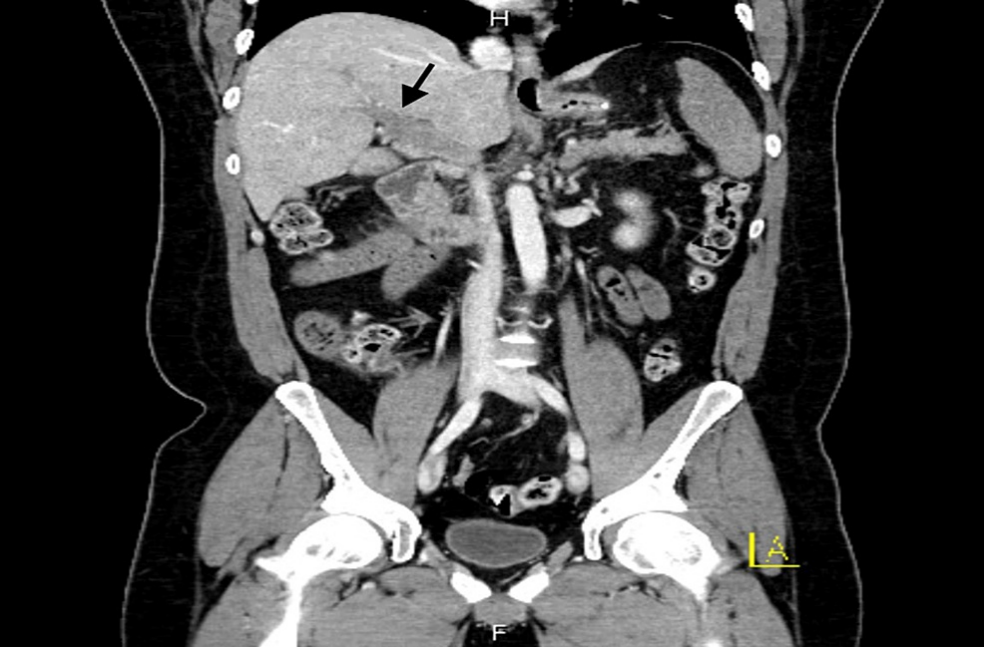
CT with contrast coronal images show nonenhancement of portal venous system consistent with complete thrombosis of portal venous system (black arrow).
